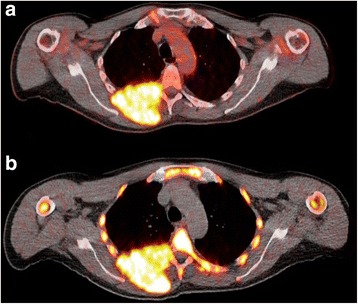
Transaxial ^18^F-FDG PET/CT (a) and ^18^F-FLT PET/CT (b) images at the thoracic level of a 60-year-old male patient with symptomatic MM. Myeloma lesion of the seventh rib with extramedullary tumor expansion to the surrounding soft tissues and thoracic vertebra after disrupting the cortical bone. The lesion shows increased accumulation in both ^18^F-FDG and ^18^F-FLT PET/CT imaging
